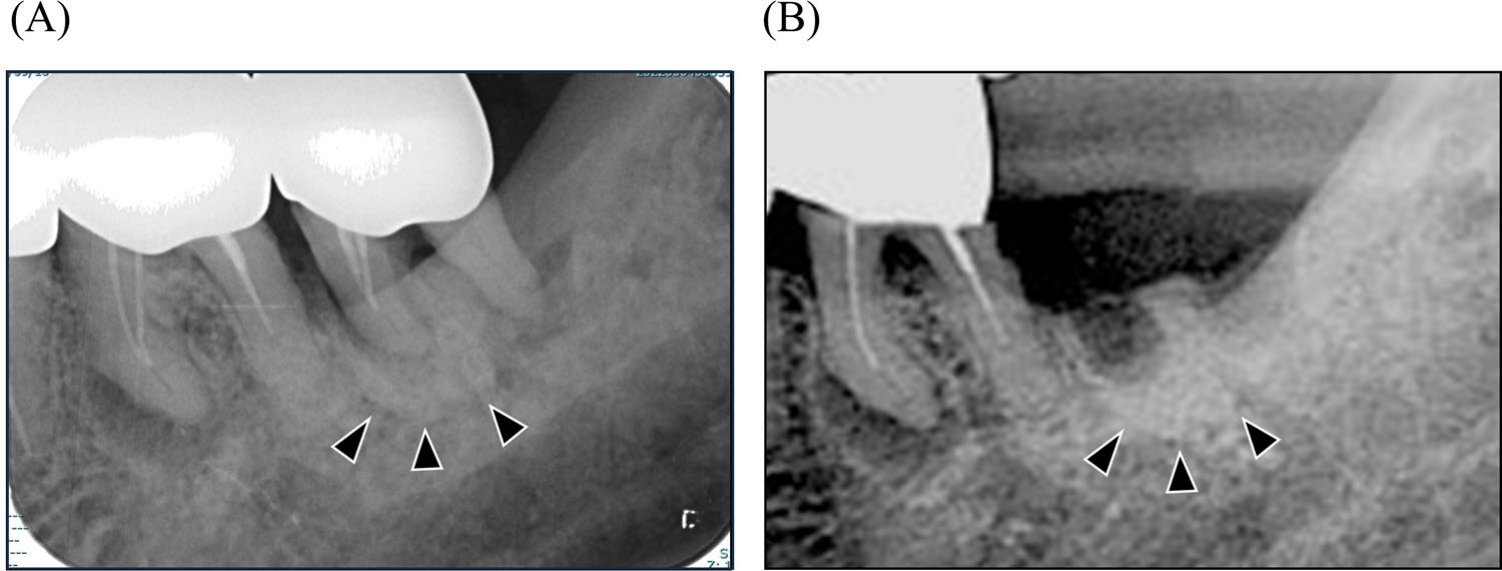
Dental image of radiopaque changes around the root. A Image before tooth extraction. B Image after tooth extraction. The black arrows indicate the part of the abnormal radiograph finding
